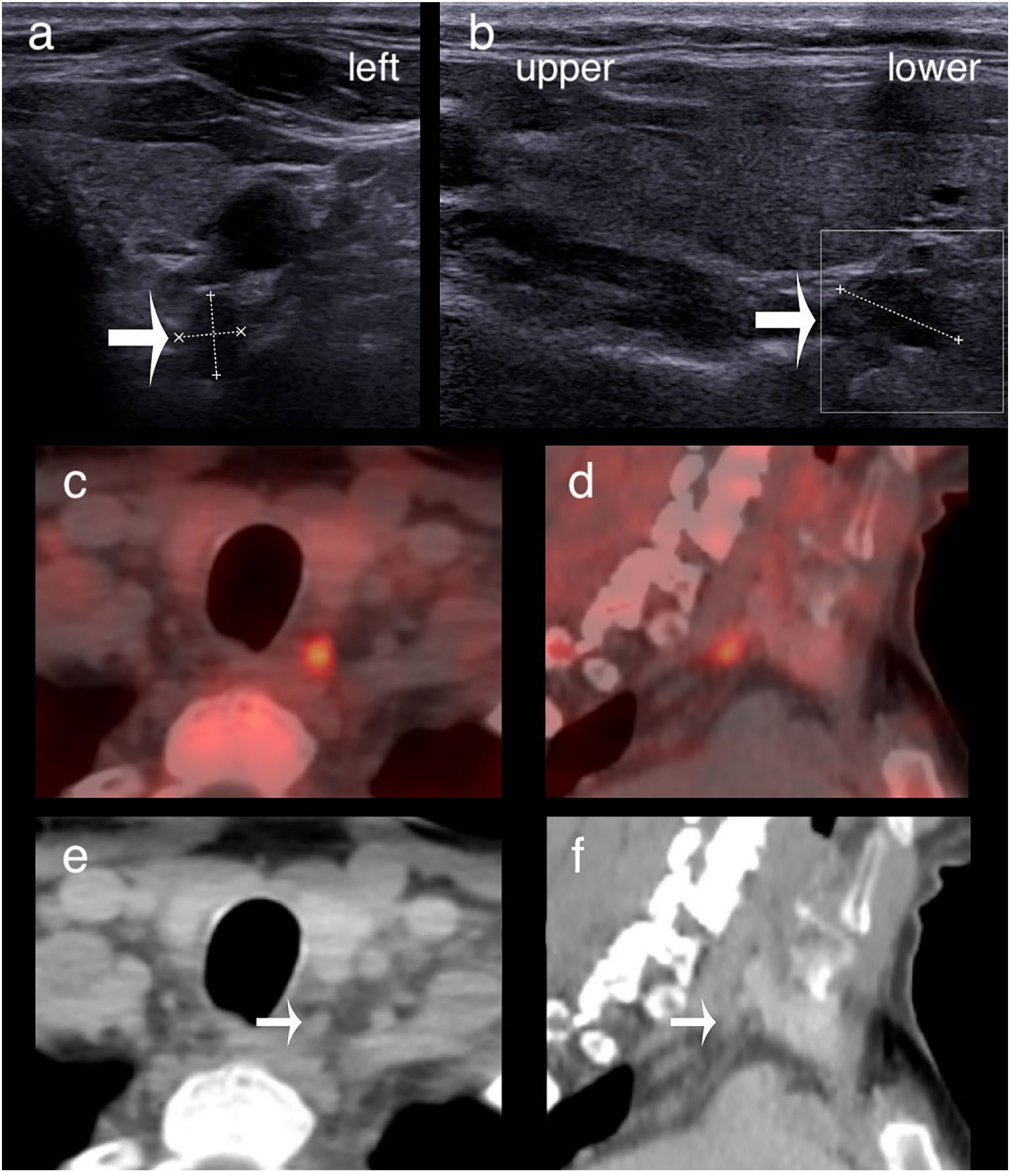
Upper parathyroid adenoma localised inferiorly by ultrasound and [^18^F]FCH PET/CT. (a,b) Transverse and longitudinal ultrasound sections. (c,d) Fused [^18^F]FCH PET/CT axial and sagittal slices. (f) Corresponding CT slices. The adenoma (arrows) is seen posteriorly to the inferior third of the left thyroid lobe but was surgically revealed to be an upper adenoma.
